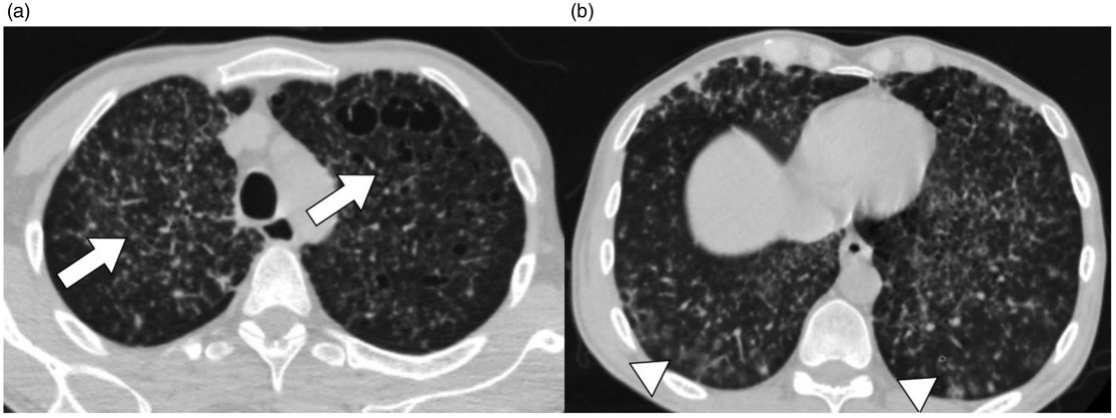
Chest CT-scan (a) Emphysema (arrow) associated with miliary-pattern infiltrates
